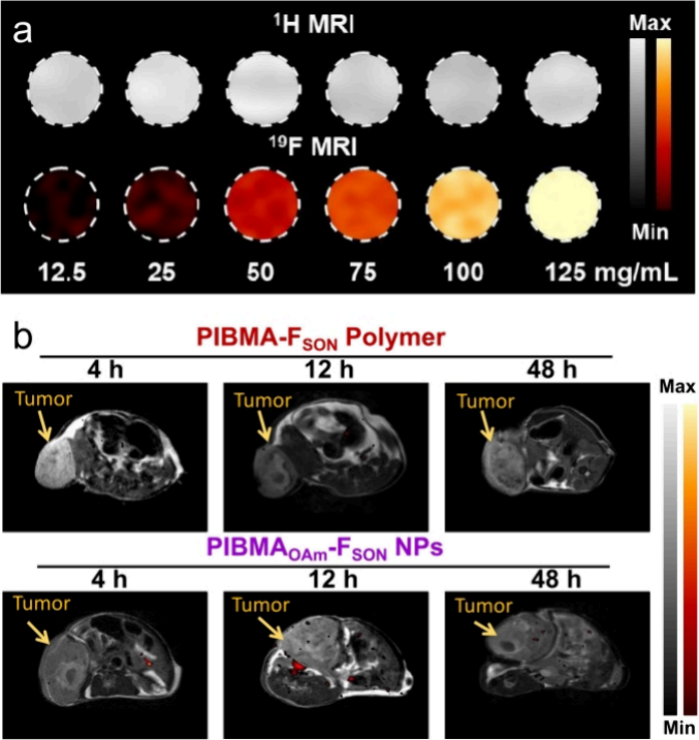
(a) ^1^H and ^19^F MRI of PIBMA_OAm_-F_SON_-IR780 NPs with various concentrations.
